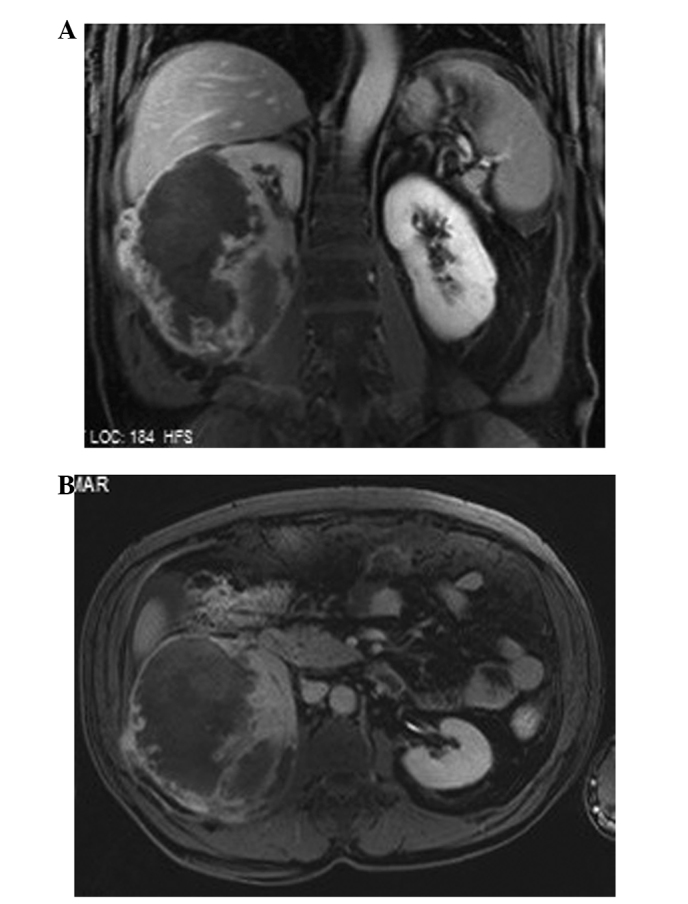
Contrast-enhanced three-dimensional magnetic resonance imaging in (A) coronal and (B) axial planes revealing a necrotic mass in the lower portion of the right kidney with no central enhancement.
